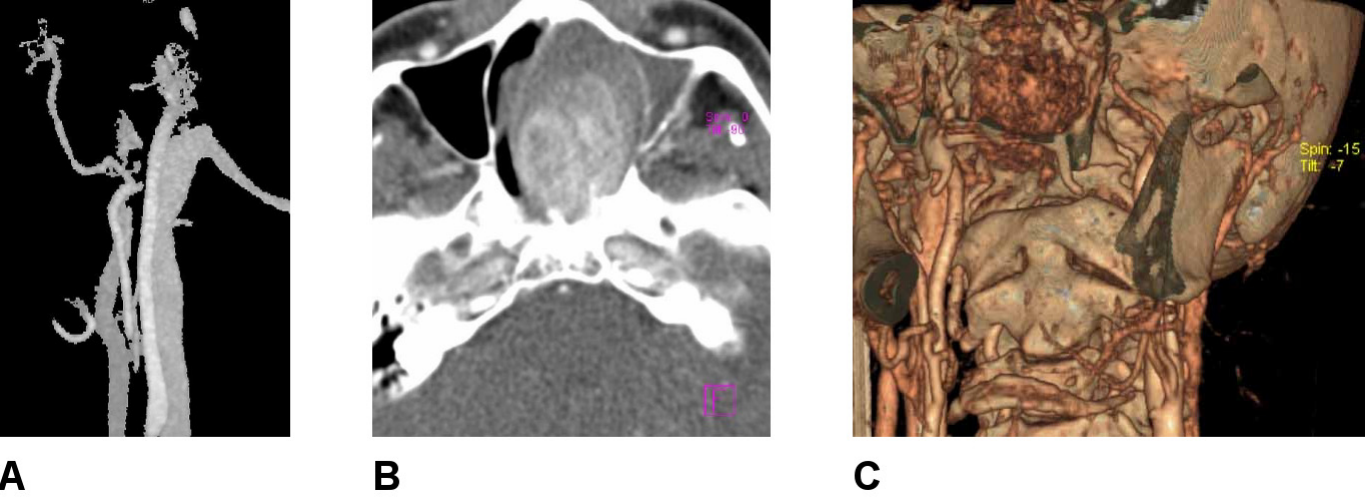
Scans of a nasopharyngeal angiofibroma. (A) Digital subtraction angiography (maximum intensity projection technique): the terminal branch of the left maxillary artery is at the hilus of the pathological angiofibroma neovascularization. (B) Computed tomography of the viscerocranium: nasopharyngeal angiofibroma seen with intravenous contrast. (C) The same tumor seen with computed tomography carotidography (volume rendering technique).
